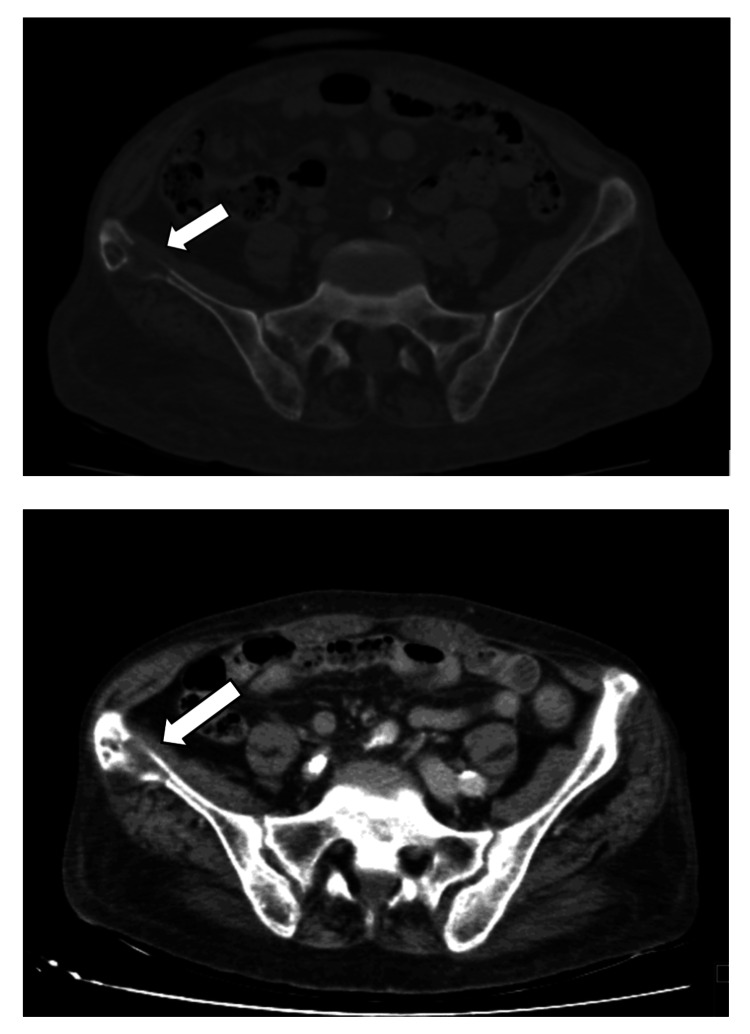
Abdominal CT scan. Transversal views of abdominal CT scan showing an osteolytic lesion in the right iliac wing (arrow), with cortical lysis in its anterior and posterior slopes. No masses in the adjacent soft tissues are visualized.
